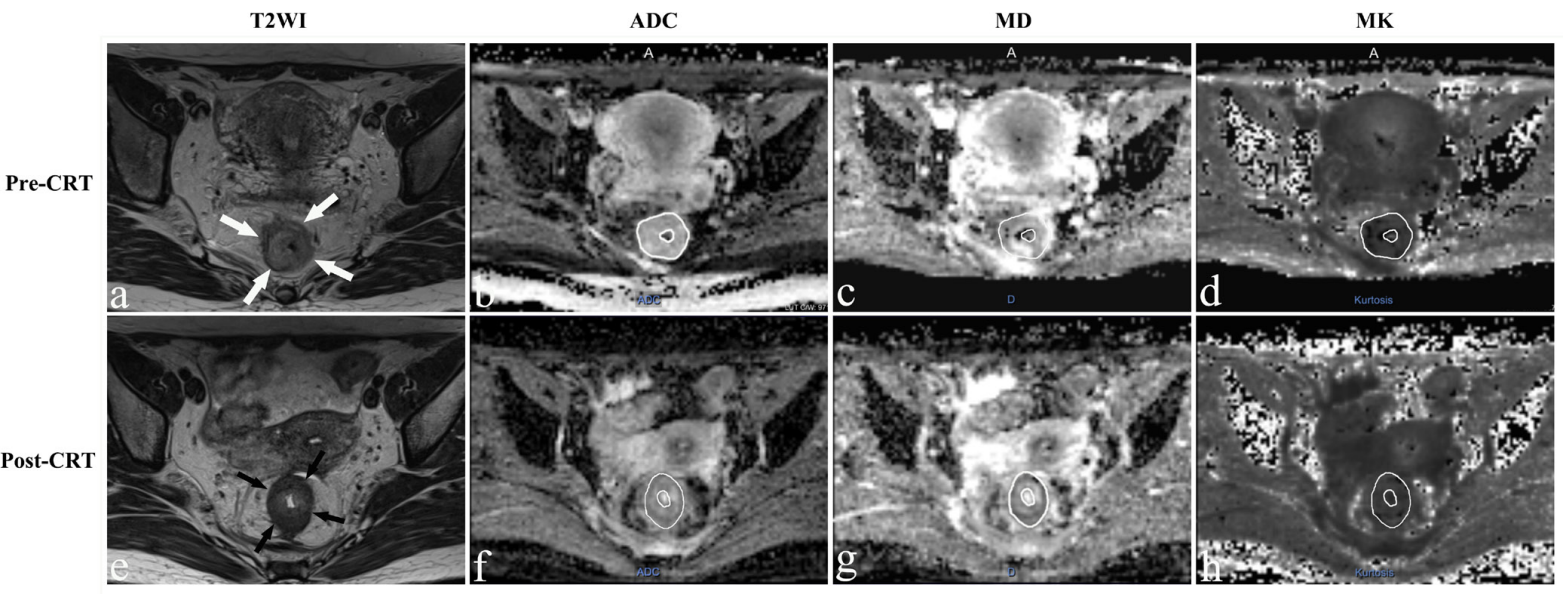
Representative images of a 36-year-old woman with a pCR Images in each row are from two measurement time points: 2-5 days before CRT (pre-CRT) and 1-4 days before surgery (post-CRT). a., e. Before and after CRT T2-weighted MR image shows that there is a tumor with ring shape visible in the rectum (arrows). The ADC map, D map, and K map have similar image contrast and manual tracing of ROIs within the tumor area (The whole tumor was not shown here). The ADC, MD and MK values were 0.932×10^-3^ mm^2^/s, 1.580×10^-3^ mm^2^/s and 0.657 before treatment. The ADC and MD values increased obviously to 1.240×10^-3^mm^2^/s and 2.601×10^-3^mm^2^/s, while the MK value decreased slightly to 0.535 after CRT.
